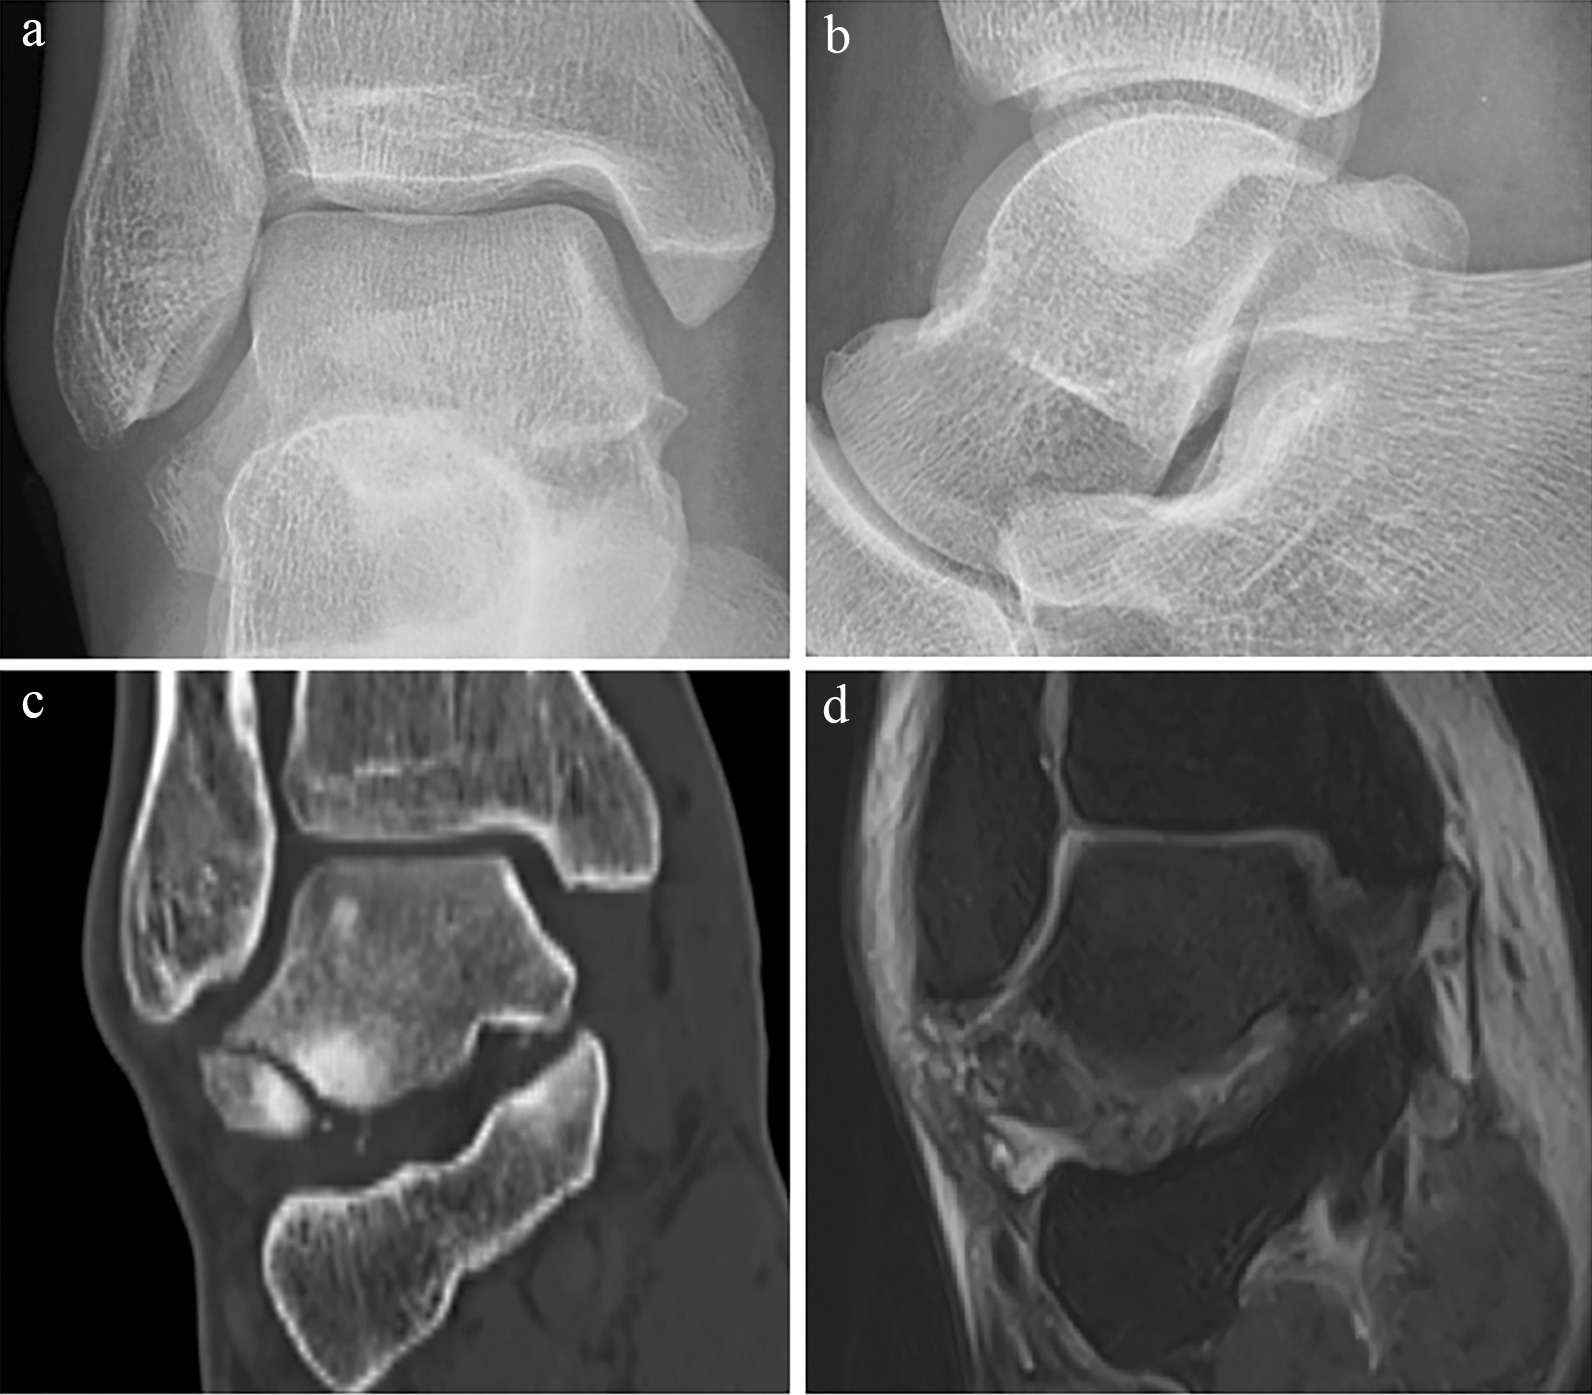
The preoperative imaging data of case 1. a Anteroposterior and b lateral radiograph of the injured ankle showed obscure fracture lines of the lateral process of the talus. c CT scan revealed fracture of the lateral process of the talus as well as soft tissue swelling around the medial malleolus. d MRI using fat-suppressed proton-density turbo-spin-echo showed high signal around the superficial deltoid ligament and mixed signal of the deep deltoid ligament
